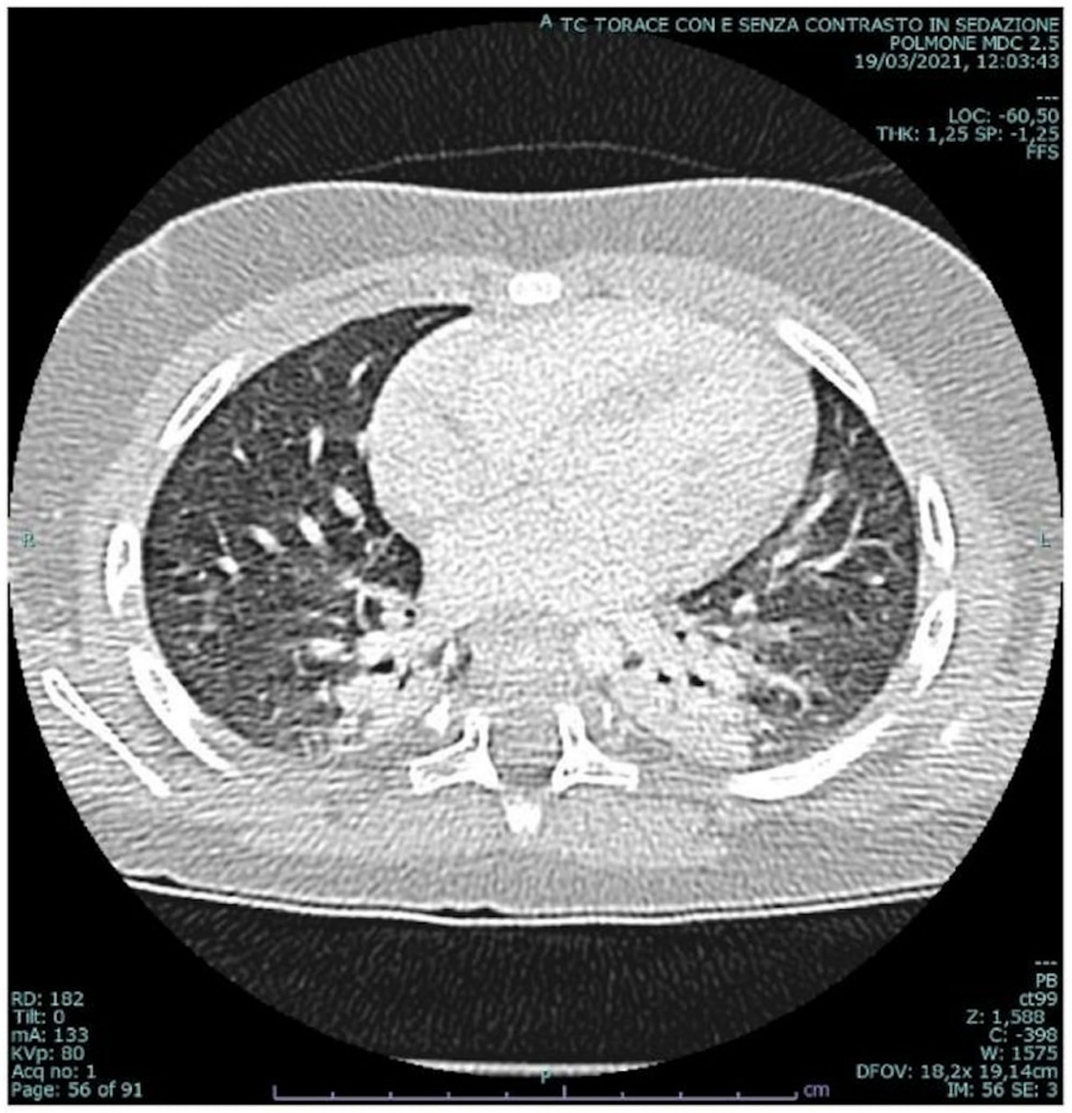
High resolution CT bilateral consolidations in the apical segment of the lower lobes with air bronchogram
